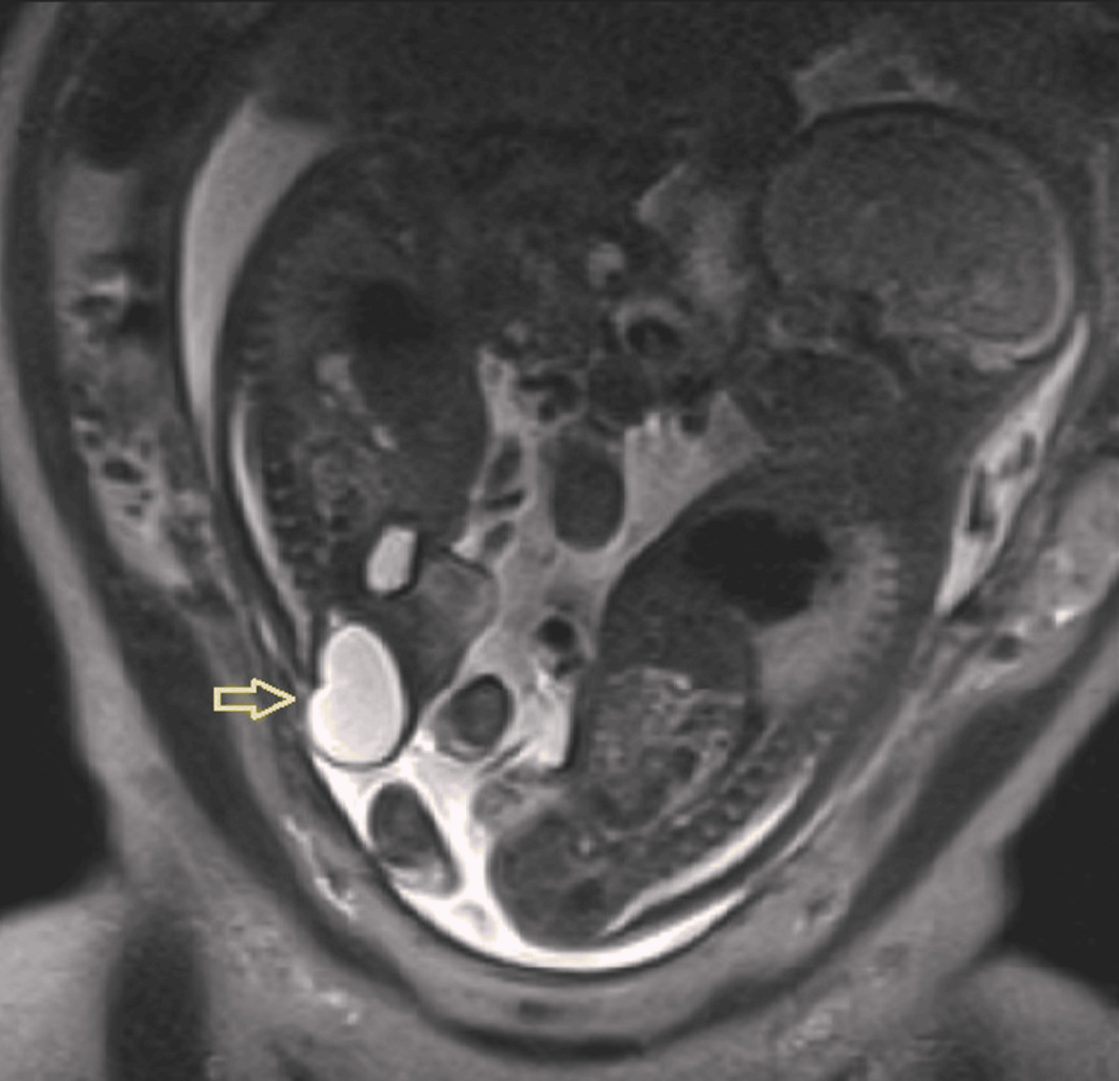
MRI (sagittal plane) at 26 weeks showing the cystic formation (arrow) in the affected fetus
